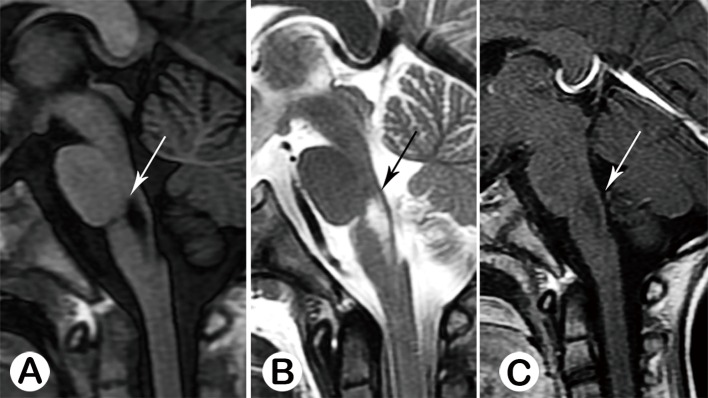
Convalescence Stage, Male, 4 years old, on the 28th day of the disease. MR images showed there is a longitudinalmalacia cavity at the posterior junction region of the pons and medulla, with prolonged T1 (A, white arrow) and prolonged T1 (B, black arrow) signal, without enhancement (C, white arrow). This subject had had impaired function of breath and recurrent pneumonia after discharge.
